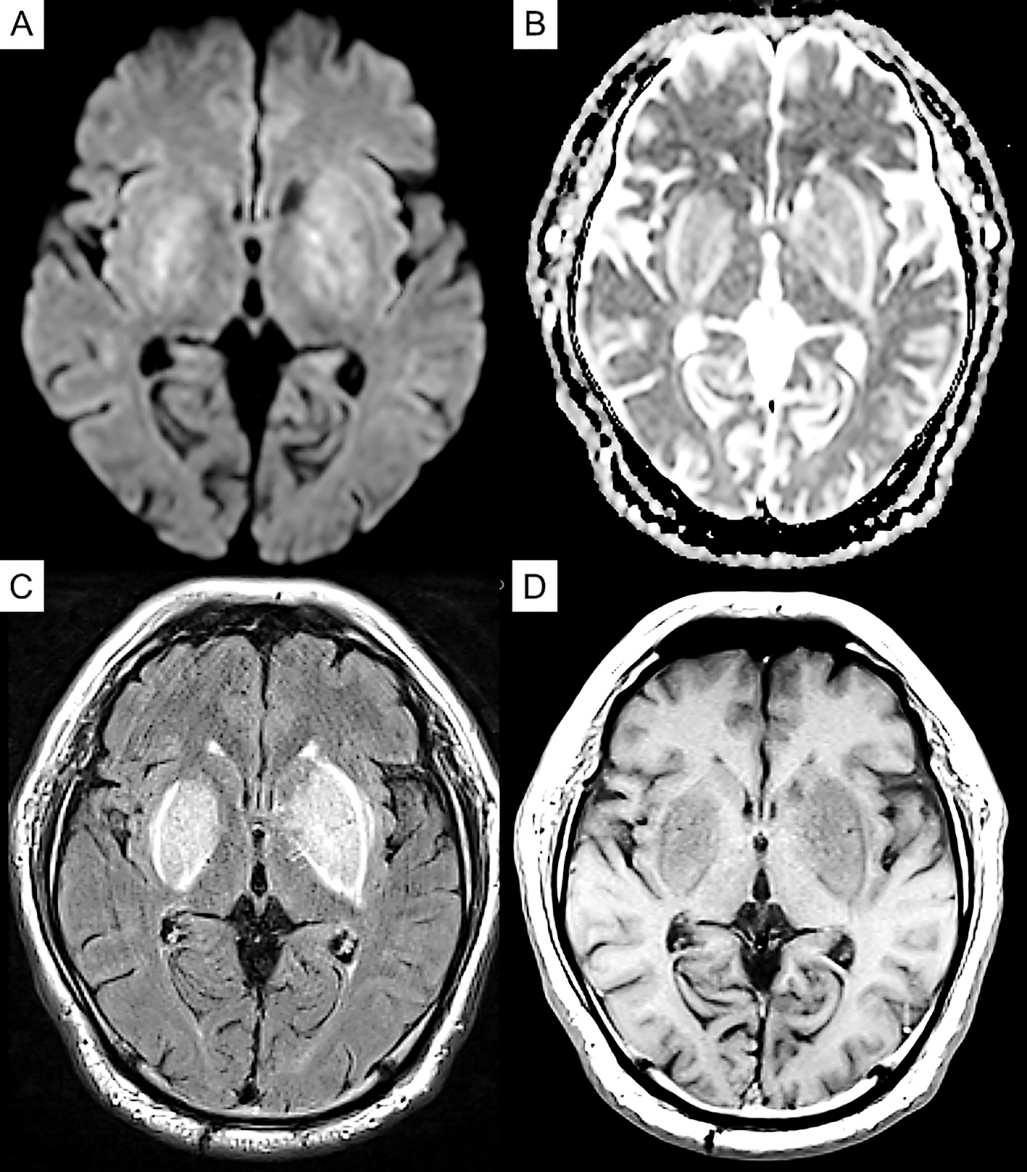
Axial brain magnetic resonance imaging (MRI) on admission. (A) Diffusion-weighted image revealing mild symmetrical restricted diffusion over both basal ganglia. (B) The lesion was hyperintense on the apparent diffusion coefficient map. (C) Fluid-attenuated inversion recovery image showing hyperintensity in the lentiform nuclei and head of the caudate nuclei with surrounding edema in the internal, external, and extreme capsule regions. (D) T1-weighted magnetic resonance image demonstrating slight low-signal changes.
